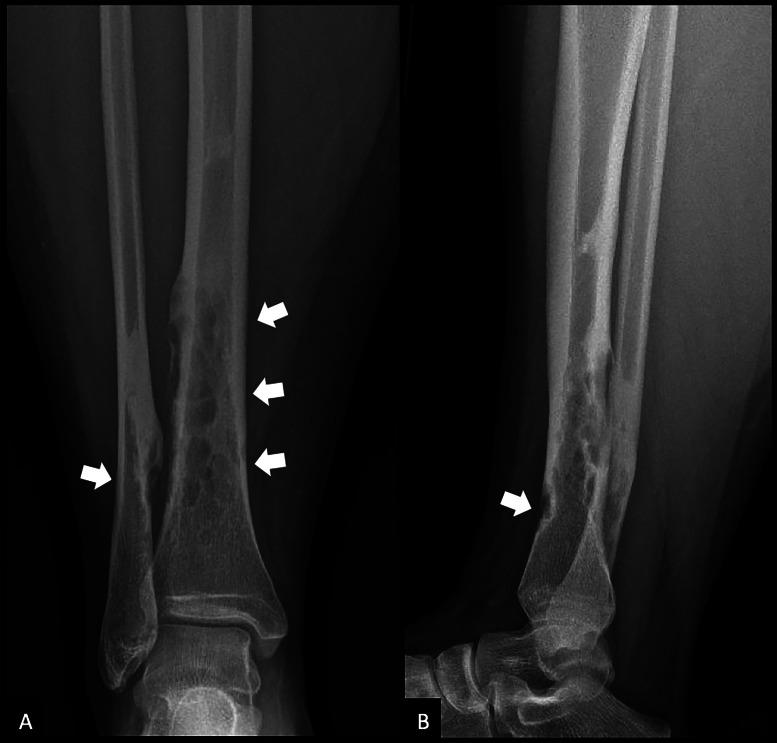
Conventional radiographs of the right leg, anteroposterior (A) and lateral (B) views. Cortical osteolytic lesions are visible in the distal third of the tibia and fibula (arrows). The lesions are well‑defined and associated with cortical thinning, without periosteal reaction or soft tissue involvement.
